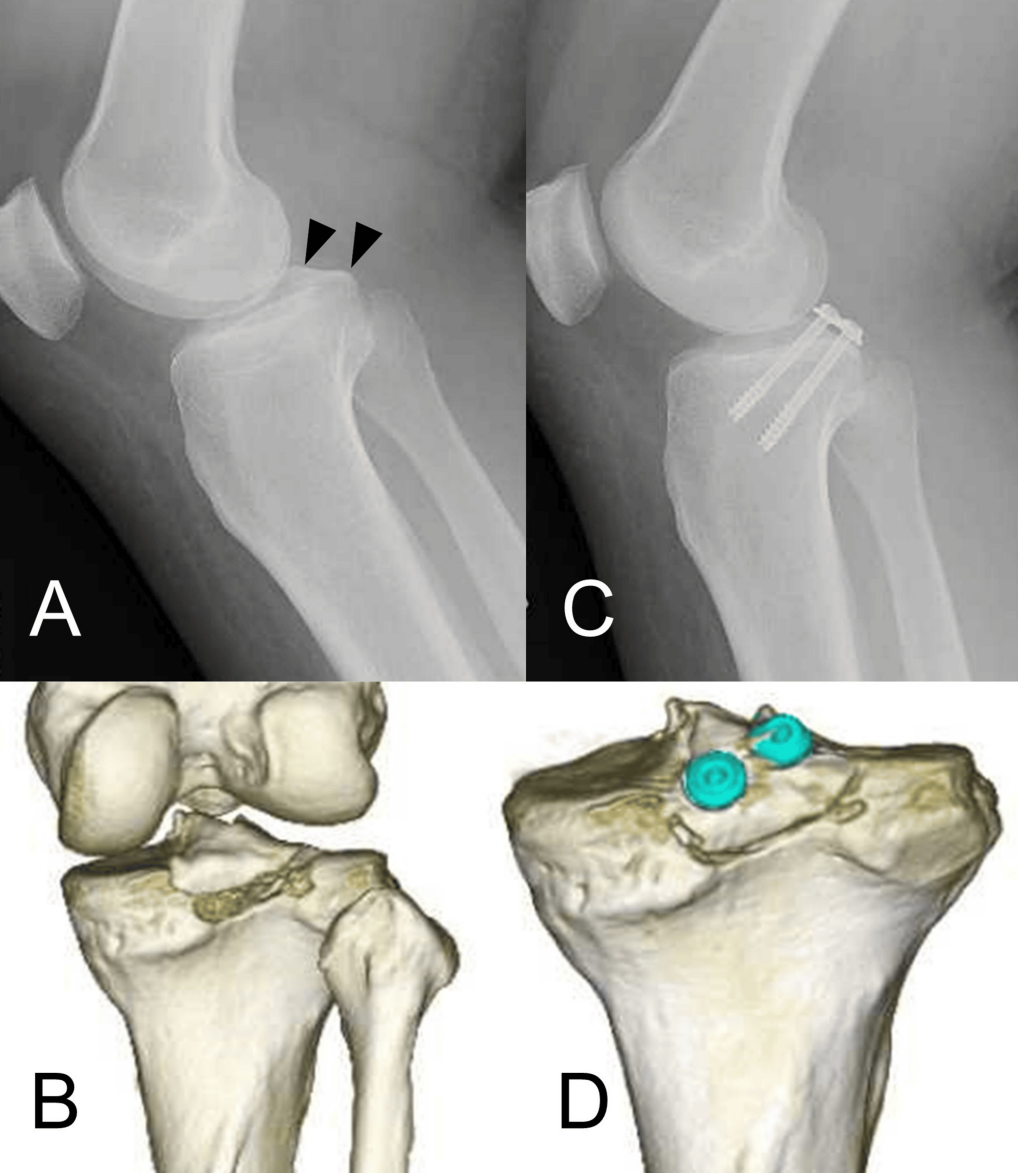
Case 1 (A) Preoperative lateral radiograph (arrowhead: bony fragment), (B) CT image. (C) Postoperative lateral radiograph and (D) CT image. CT, computed tomography; MRI, magnetic resonance imaging.
